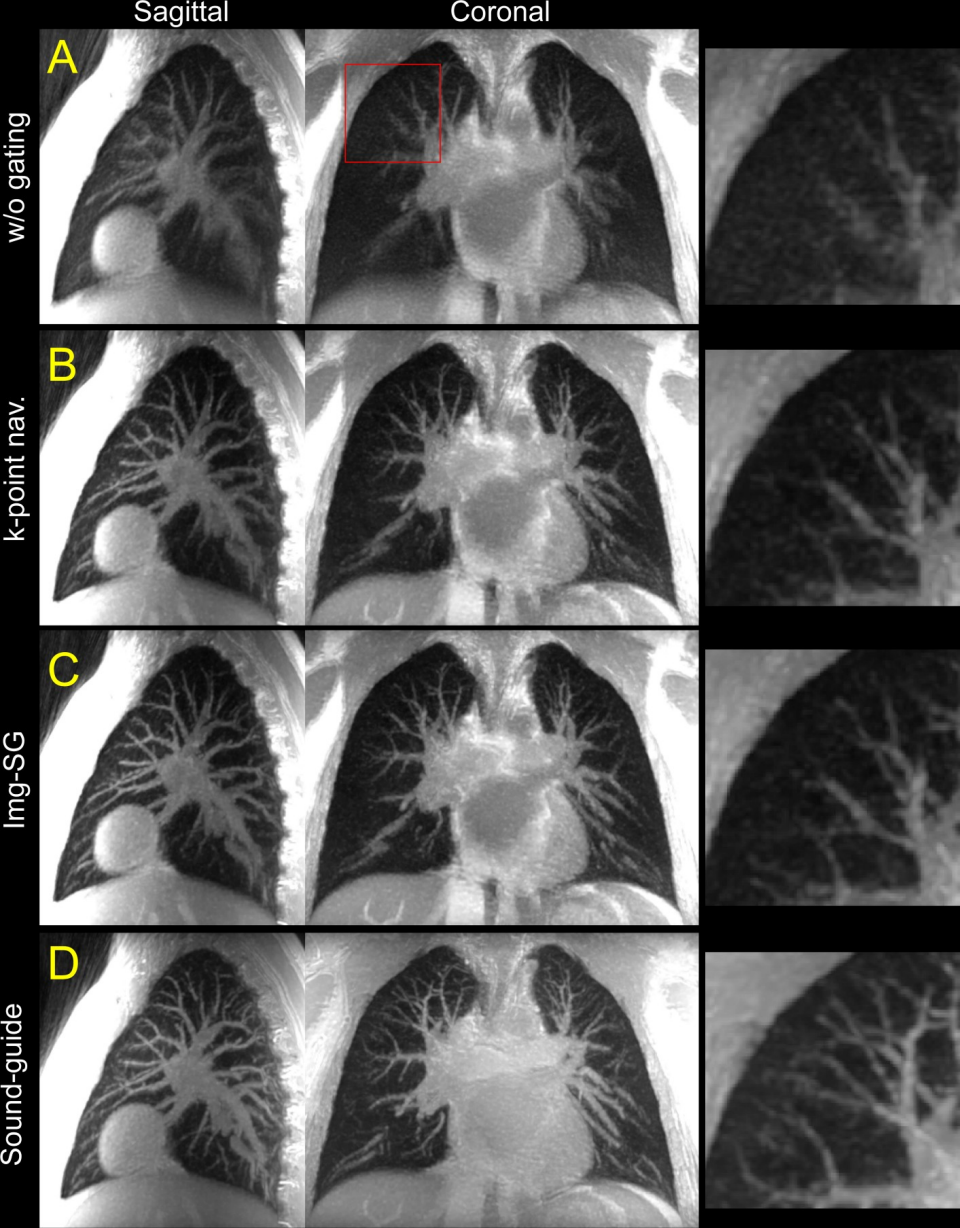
Maximum intensity projection (MIP) of high-resolution UTE MRI images. MIP images for free-breathing without respiratory motion management (w/o gating, A) and free-breathing with retrospective respiratory gating (k-point navigator, B, or image-based self-gating [Img-SG], C), and sound-guided respiration (Sound-guide, D) are presented in sagittal (left) and coronal (center) views. Images obtained with respiratory motion management (B-D) clearly show improved delineation of pulmonary vasculature as compared to w/o gating (A). Moreover, the MIP image from Sound-guide shows notably better visualization for peripheral blood vessels in zoomed views as compared to k-point navigator and Img-SG (right). The MIP images were computed from a 2.1-cm thick slab for both sagittal and coronal views. The images shown in this figure are from the same subject as the one in Figs 2–5. Coronal MIP images with a projection slab sliding from posterior to anterior are shown in S1 Video.
